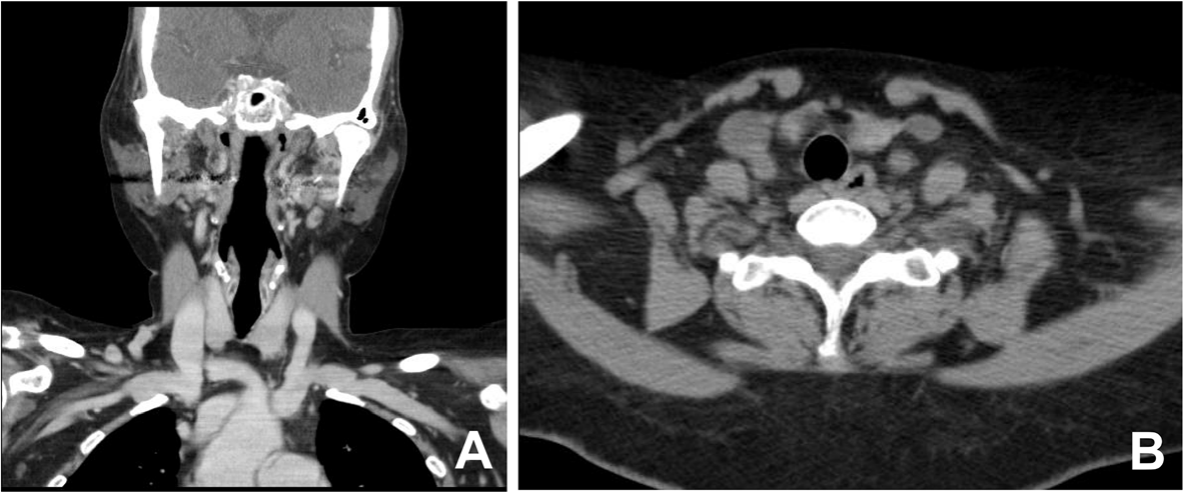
Computed tomography image of the patient’s neck. CT images were taken to evaluate the trachea and esophagus. There was no apparent airway or esophageal obstruction on the coronal view (a) or the axial view (b). CT: Computed tomography
